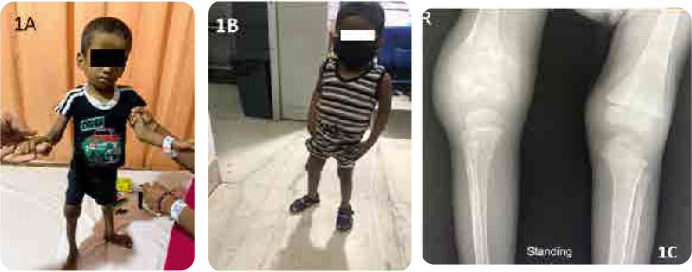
Patient 1. A. Patient 1 Emaciated, Frontal bossing, bony swelling of the right knee. B. Significant weight gain with treatment. C. Plain X-Ray of bilateral knees demonstrating persistent right joint effusion and swelling with soft tissue involvement. Abnormal widening and cupping of distal metaphyses, enlarged epiphyses with trabeculations.
